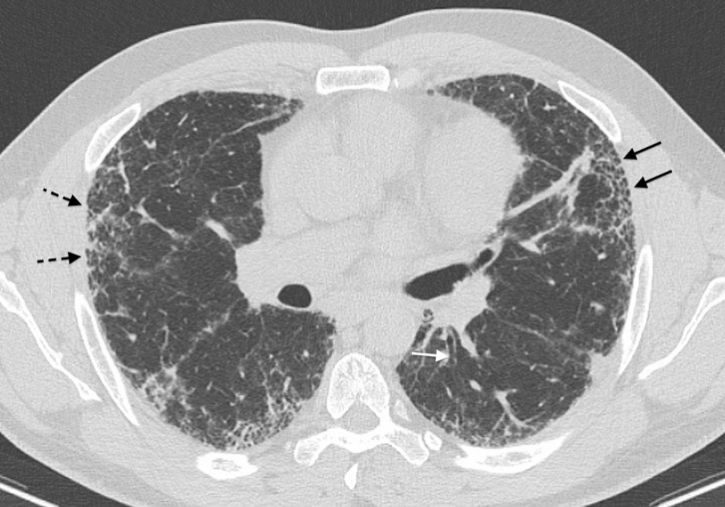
HRCT image of a UIP pattern (same patient of Fig. 1). Typical imaging features of UIP are clearly recognisable because of the presence of honeycombing (black arrows), traction bronchiectasis (white arrow) and a reticular pattern (black dotted arrows). Lesions are typically located along subpleural regions
